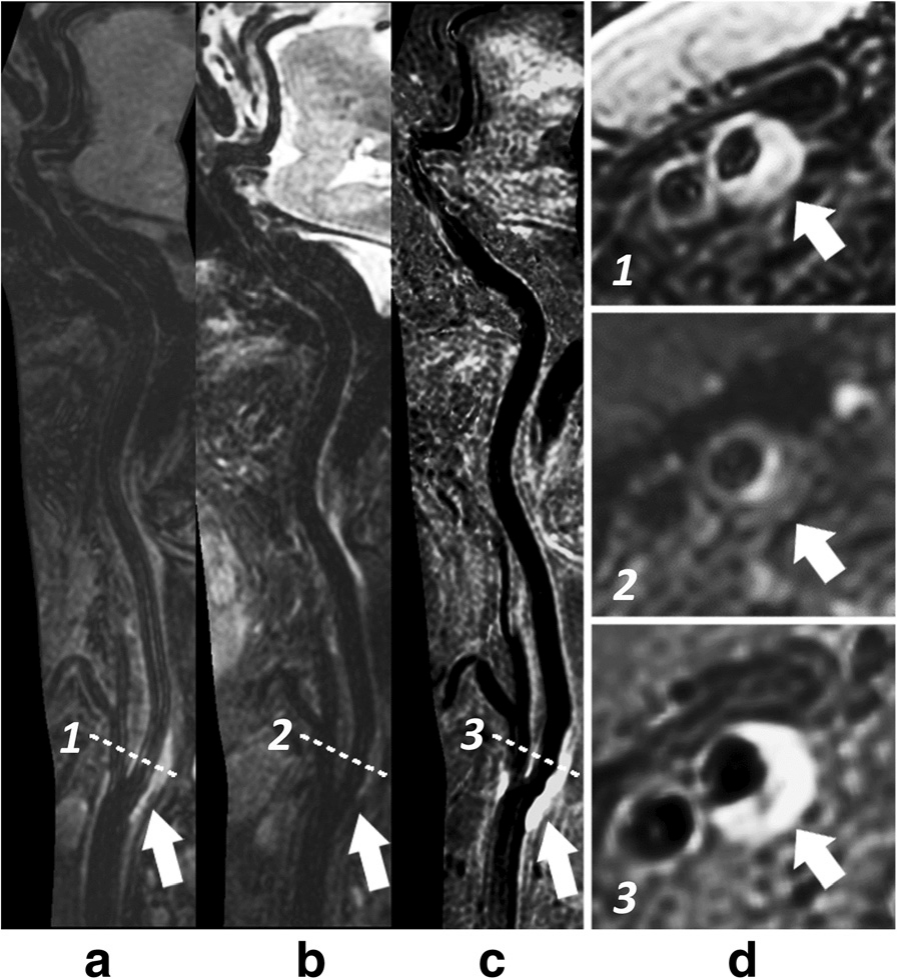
Visualization of one local plaque in 3D-MERGE, T2-weighted VISTA and SNAP images. Both curved longitudinal and cross-sectional views of the intracranial and carotid arterial walls can be observed using MPR on 3D-MERGE (a), T2-weighted VISTA (b) and SNAP (c) images. The locations of the 3 cross-sectional images in (d) are marked by the dashed lines on the curved longitudinal images. IPH component and severe wall thickening distributed around the carotid bulb can be confirmed based on the 3D multi-contrast images
